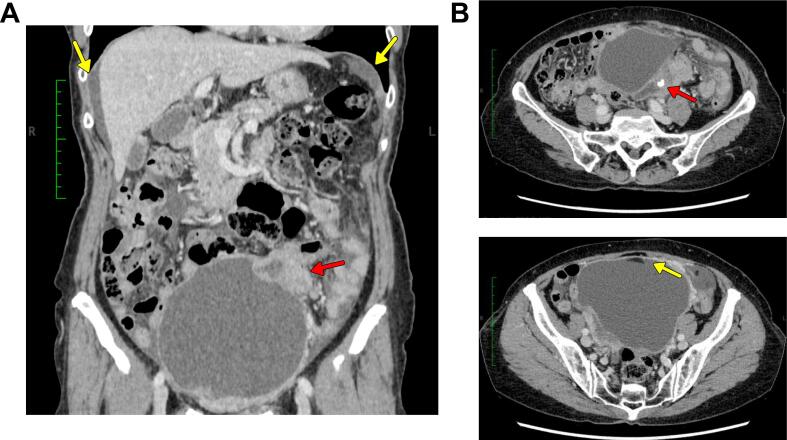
Computed tomography (CT) imaging before surgery. A Coronal CT image. A 10-cm-sized pelvic tumor with a solid part is detected (red arrow). Ascites extending to the upper abdomen is also detected (yellow allow). B Axial CT images. The tumor lacks turgidity and is suspected to have ruptured. Fatty components and calcifications are detected within the tumor. (For interpretation of the references to colour in this figure legend, the reader is referred to the web version of this article.)
